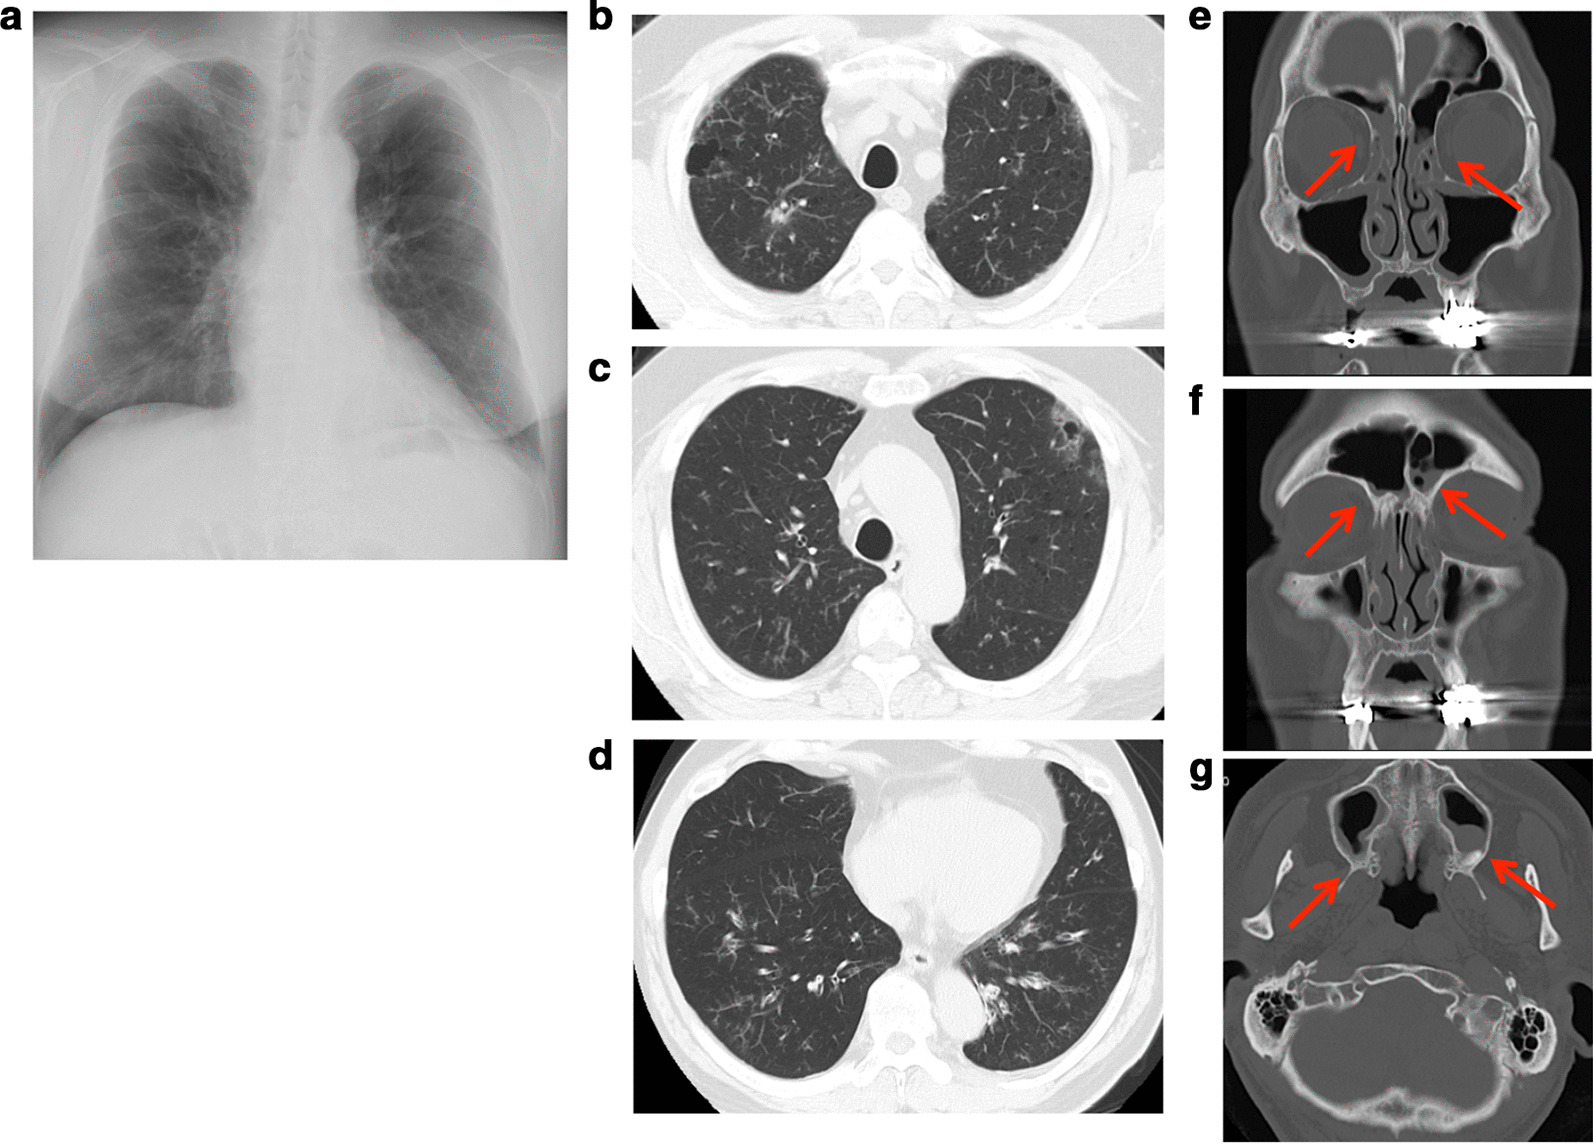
Thoracic radiogram and computed tomography (CT) scan at the bronchoscopy exam. Chest radiogram (a) showed bilateral linear shadow. Thoracic CT scan of upper (b, c) and lower lobes (d) showed bilateral ground-glass opacity. e–g Paranasal sinus CT scan; Ethmoid sinus (e), frontal sinus (f) and maxillary sinus (g). Each arrow indicates nasal mucosa thickening and fluid collection in the sinus. These appearances suggest paranasal sinusitis
